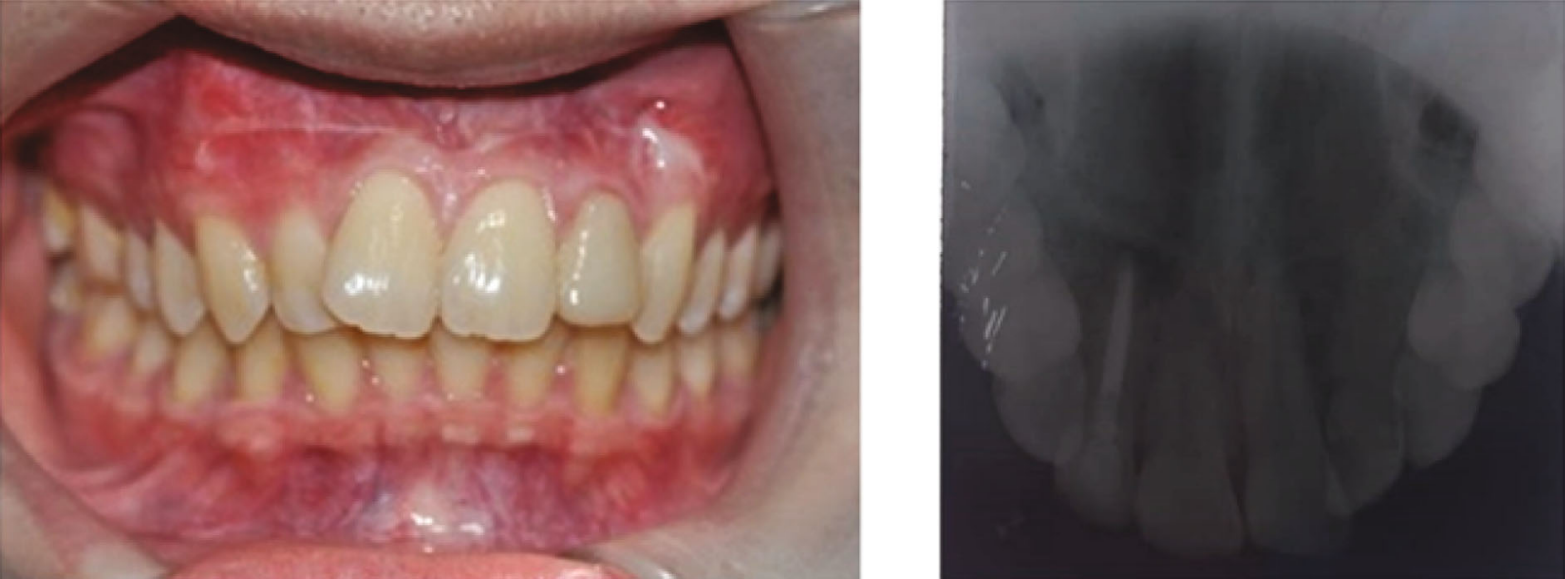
One year follow-up in radiograph with patient's tooth color appearance.
